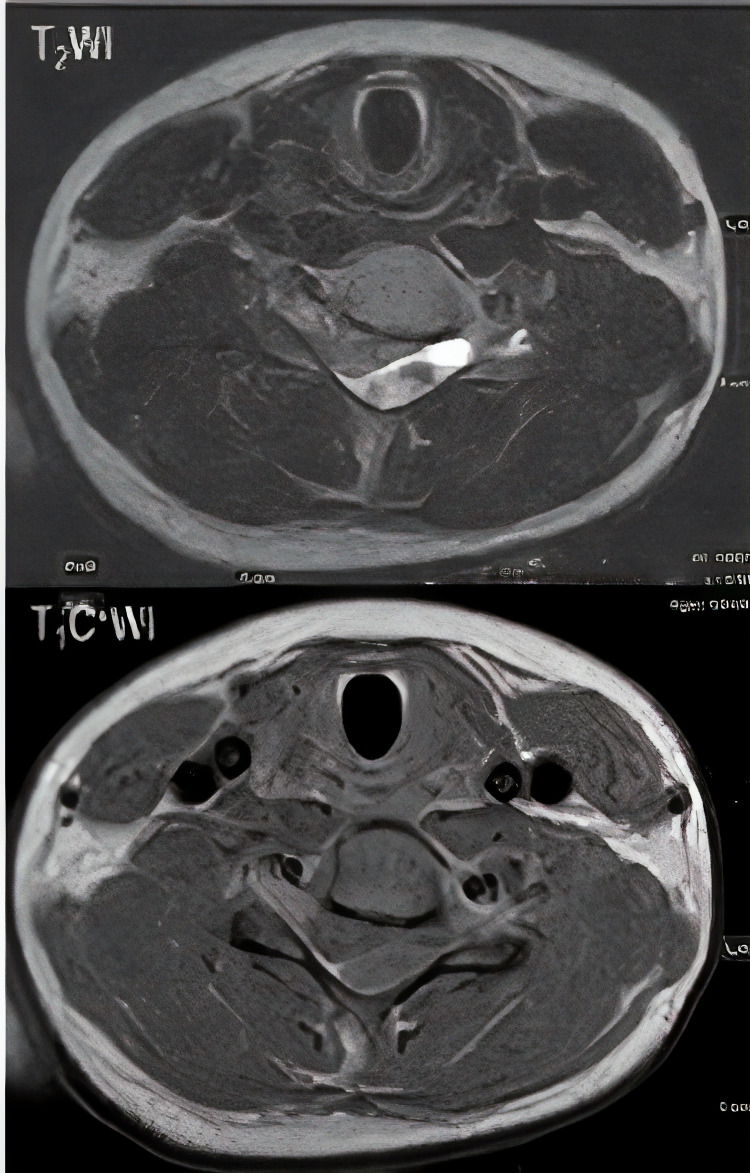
Axial MR images at same level in T2WI & T1 post contrast enhancement A heterogenous fusiform left posterolateral epidural lesion that appears heterogeneously high on T2W images with no remarkable contrast enhancement in the post gadolinium T1 study. Findings are in keeping with epidural hematoma.
